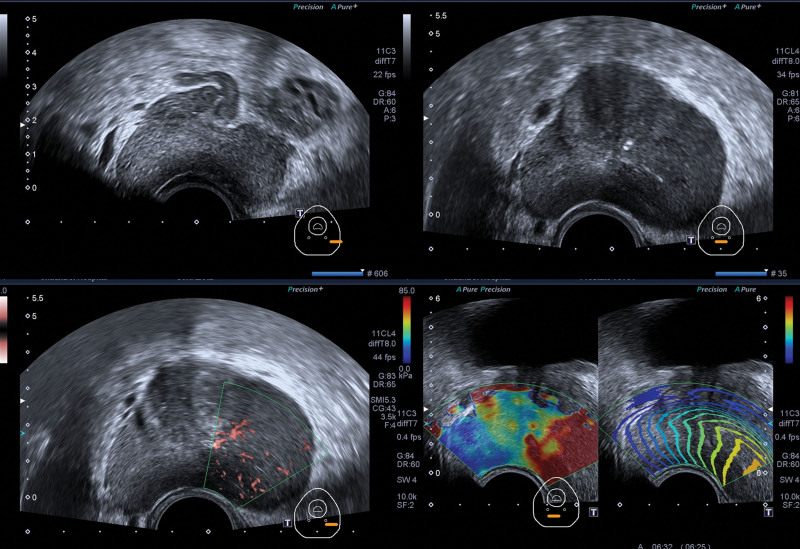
TRUS presentation: (A) The volume of the peripheral zone of the prostate was enlarged, the left peripheral zone was fuller than the right, and the gray-scale images were less uniform. (B) Color Doppler showed blood flow signal was of left peripheral zone Adler grade I. (C) Involvement of the left vesicular gland. (D) SWE showed the left peripheral zone was hard and displayed red, and the right peripheral zone was softer and displayed blue. TRUS = transrectal ultrasound.
